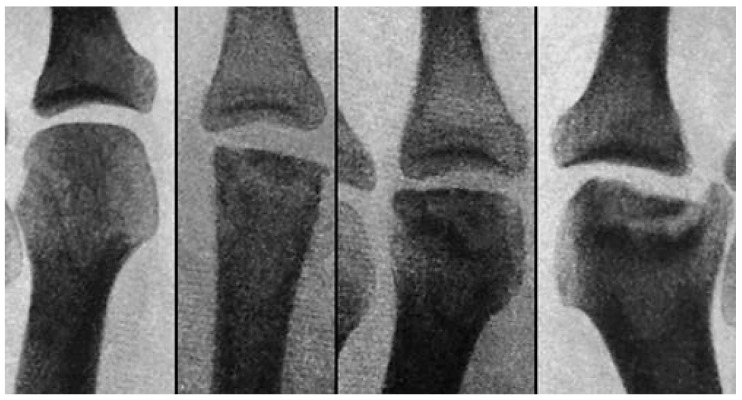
The radiographic progression of the second metatarsal head osteochondrosis in a 22-year-old female patient who had not received any treatment.
